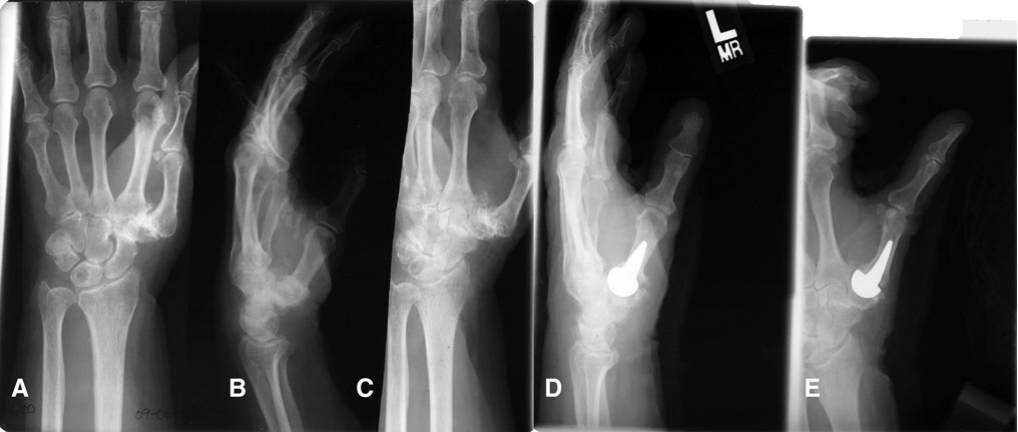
Preoperative (A) posteroanterior, (B) lateral, and (C) oblique radiographs show the left hand and wrist of a 63-year-old woman with Eaton-Littler Stage III arthritis of the basal joint of the thumb. (D) Lateral and (E) posteroanterior radiographs show the left hand and wrist 72 months after implant arthroplasty of the left thumb basal joint.
